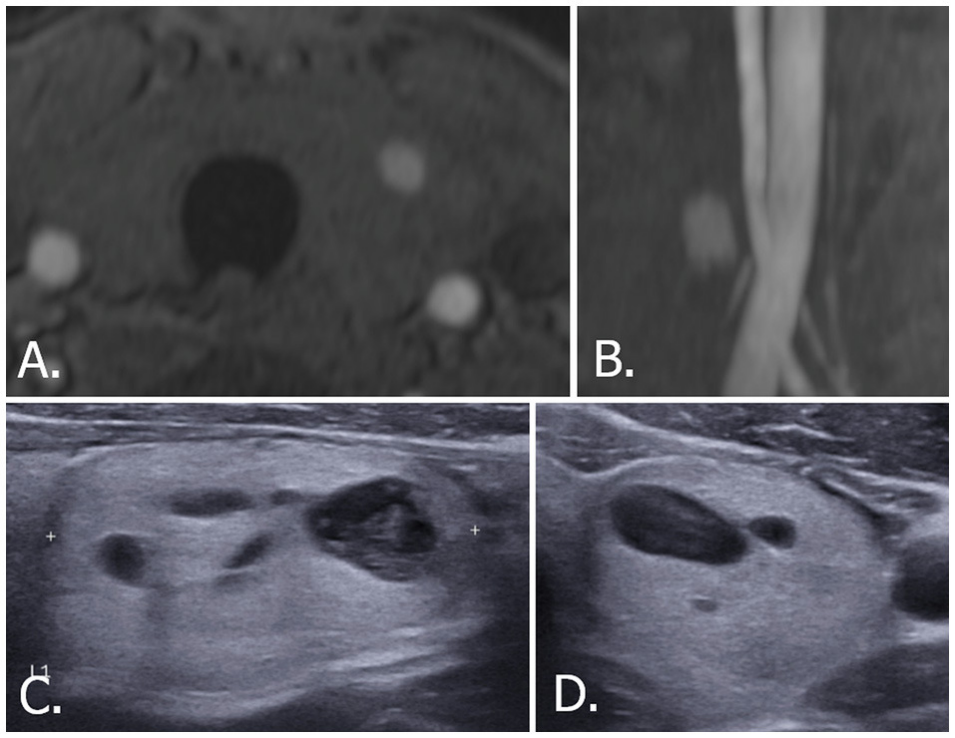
70-year-old male with left thyroid nodule. Source (A) and MIP (B) images of TOF-MR angiography show a nodule with hyperintense portion in the left thyroid gland. Transverse (C) and longitudinal (D) US images of left thyroid demonstrate a smooth, oval, isoechoic, predominantly solid nodule, which is classified as K-TIRADS category 3, ACR-TIRADS category 2, and EU-TIRADS category 3. FNA cytologic examination revealed a benign result. The hyperintense portion on TOF-MR angiography corresponds to the cystic portion of the nodule on US images. MIP, maximum intensity projection; TOF-MR, time-of-flight magnetic resonance; US, ultrasound; K-TIRADS, Korean Thyroid Imaging Reporting and Data System; ACR-TIRADS, ACR Thyroid Imaging Reporting and Data System; EU-TIRADS, European Thyroid Imaging Reporting and Data System; FNA, fine needle aspiration.
